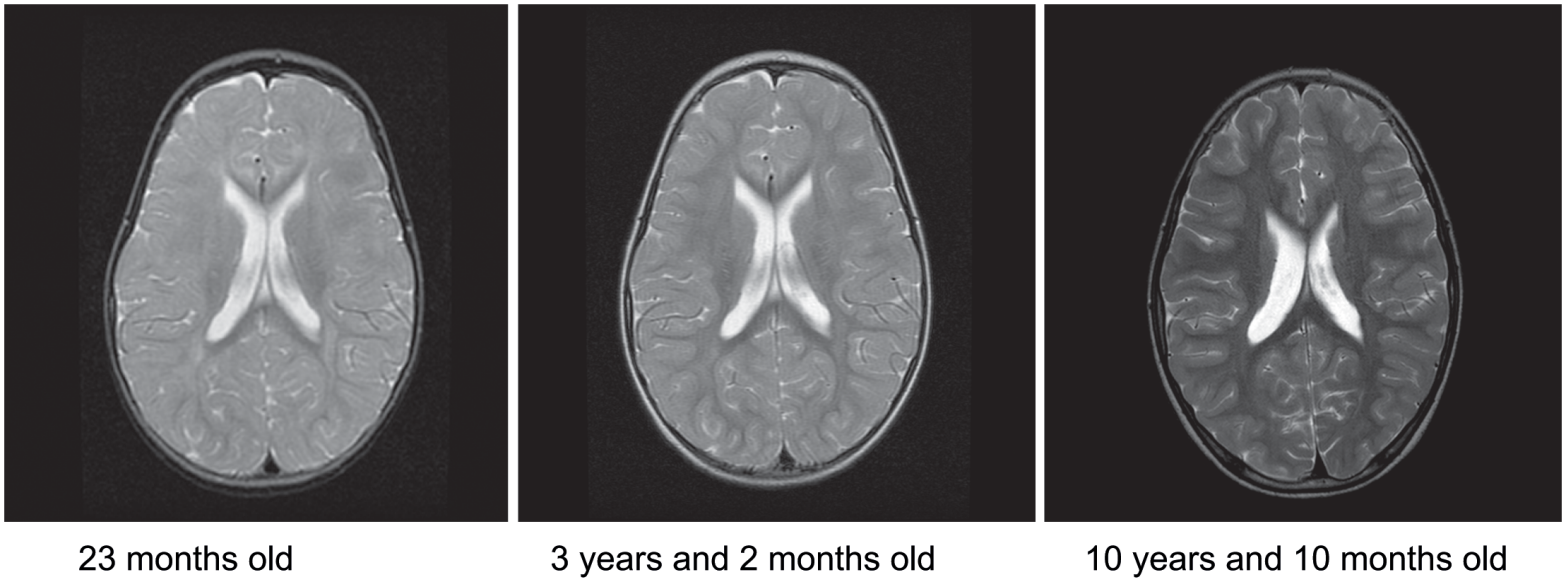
Brain MRI of the index patient. T2-weighted axial images at 23 months, 3 years and 2 months showing delayed myelination, and at 10 years and 10 months myelination has normalized.
